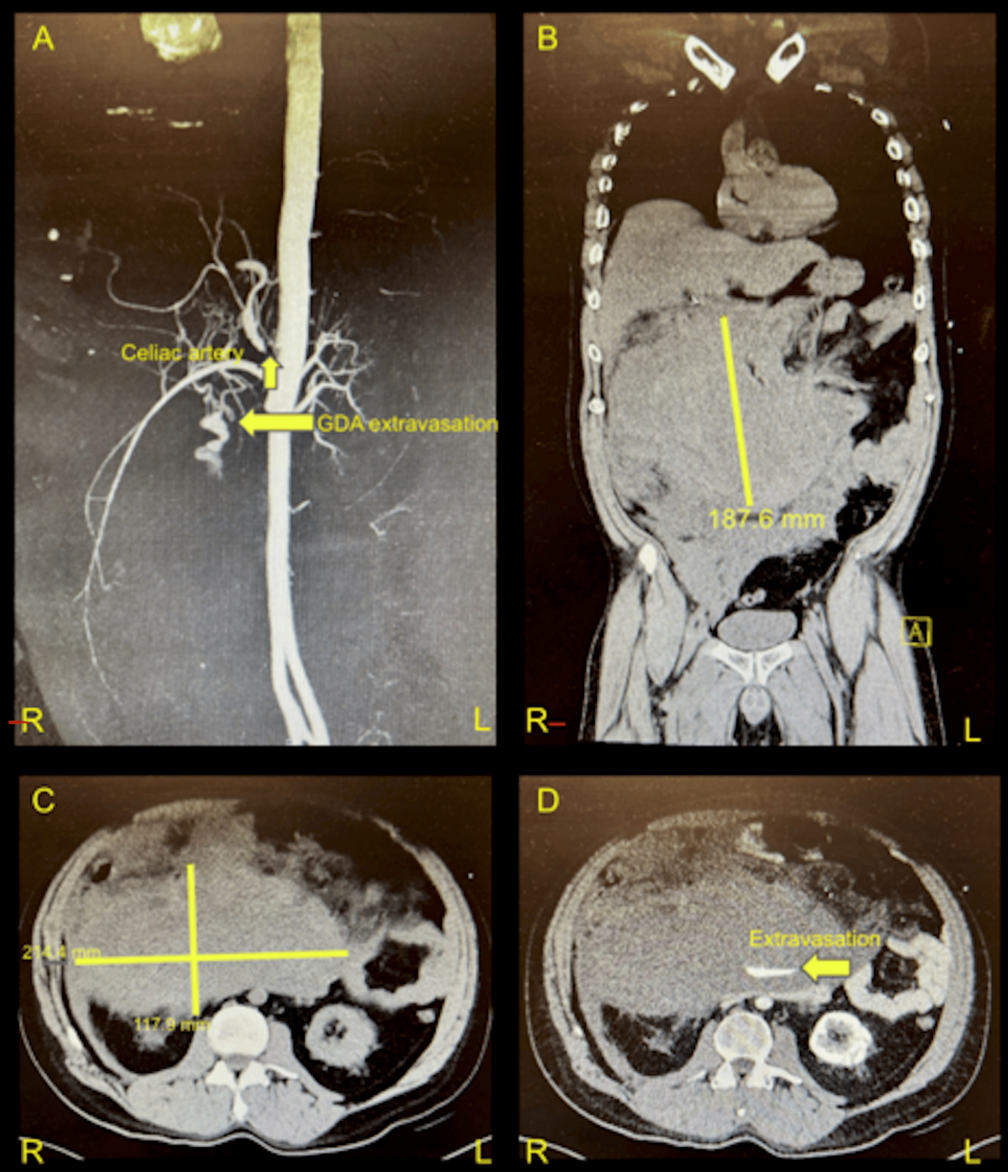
CTA of the abdomen and pelvis. (A) Vascular reconstruction demonstrating celiac trunk and active arterial extravasation. (B) Coronal view demonstrating initial hematoma with longitudinal expanse of 187.6 mm. (C) Axial view demonstrating hematoma with width of 214.4 mm and depth of 117.9 mm, approximate volume of 4.7 L. (D) Active contrast extravasation demonstrating hemorrhage into the posterior-medial aspect of the hematoma. CTA: computed tomography angiography
